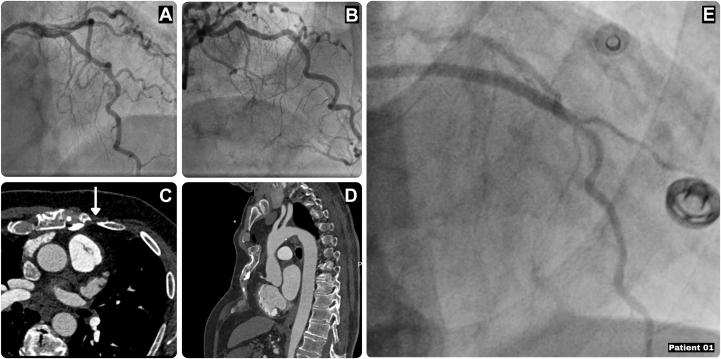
Characteristics of Patient 1 (A and B) Preoperative angiography. (C) Preoperative computed tomography with the bony overgrowth. The arrow denotes the place where the LIMA should be expected. (D) Preoperative computed tomography showing the sternum after 2 repairs for pectus excavatum. (E) Postoperative result with the SVG-LAD graft in situ. LAD = left anterior descending artery; LIMA = left internal mammary artery; SVG = saphenous vein graft.
